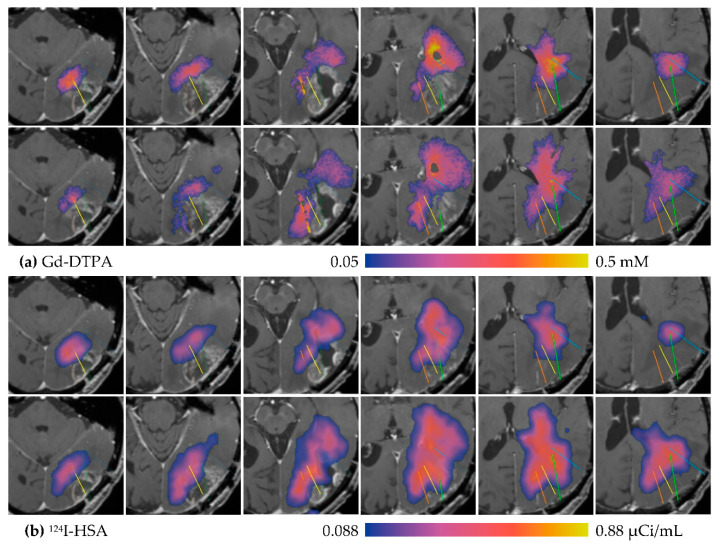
MR1-1 Patient 7 measured distribution (in color, overlaid on pre-infusion T1-weighted MRI, six axial slices, 10 mm apart). Colored lines indicate the approximate catheter placements, which are not entirely in-plane. The opaque portion of the line is inferior to the slice and the translucent portion is superior. (a) Measured Gd-DTPA concentration: (Top) after 24 h of infusion; and (Bottom) after 72 h of infusion. (b) Measured 124I-HSA concentration: (Top) after 24 h of infusion; and (Bottom) after 72 h of infusion.
